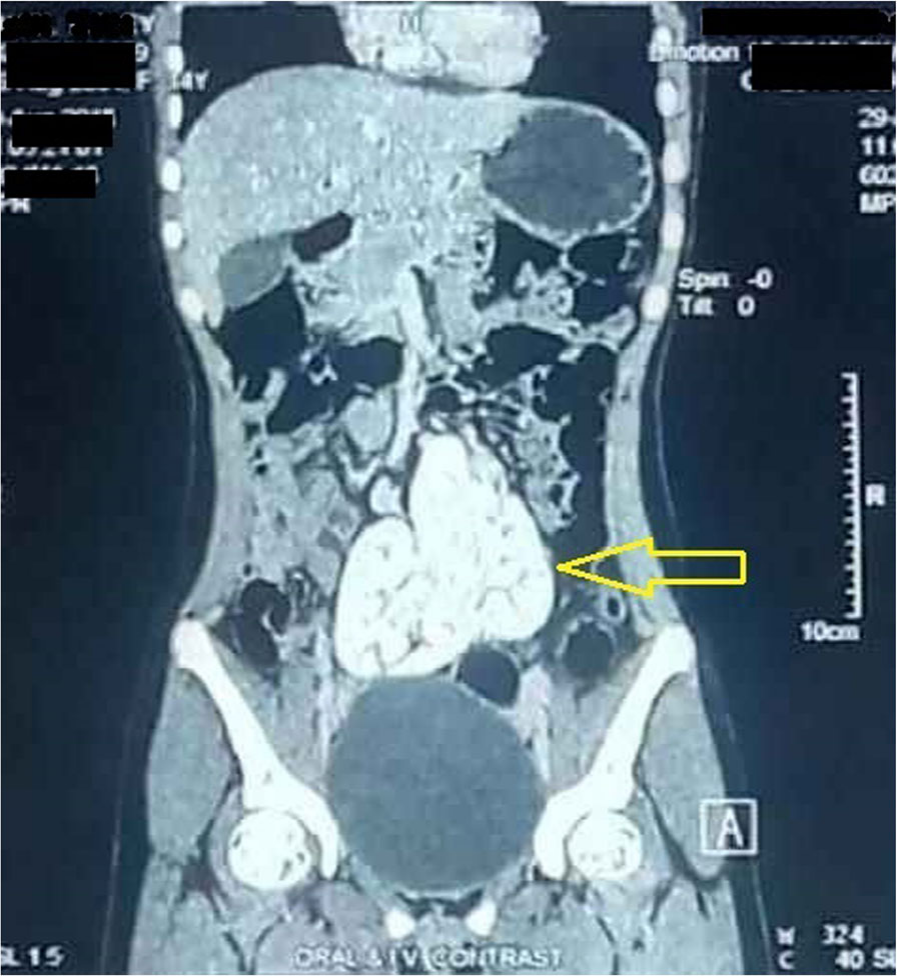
Contrast-enhanced computed tomography (multi-detector computed tomography) scan of abdomen shows pancake kidney, which is placed below the aortic bifurcation in the pelvis with short ureters (*yellow arrow* in coronal section)
